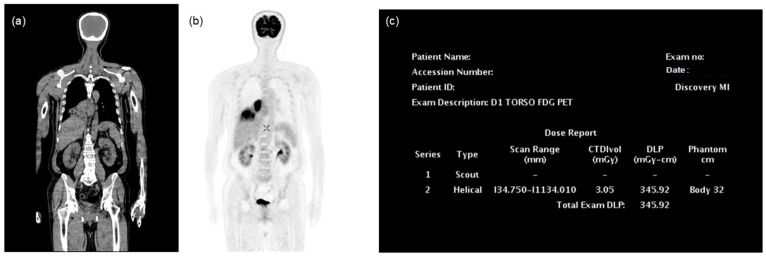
Representative images of PET/CT and a CT dose report from a patient with lung cancer. (a) coronal CT (b) coronal PET (c) CT dose report.
